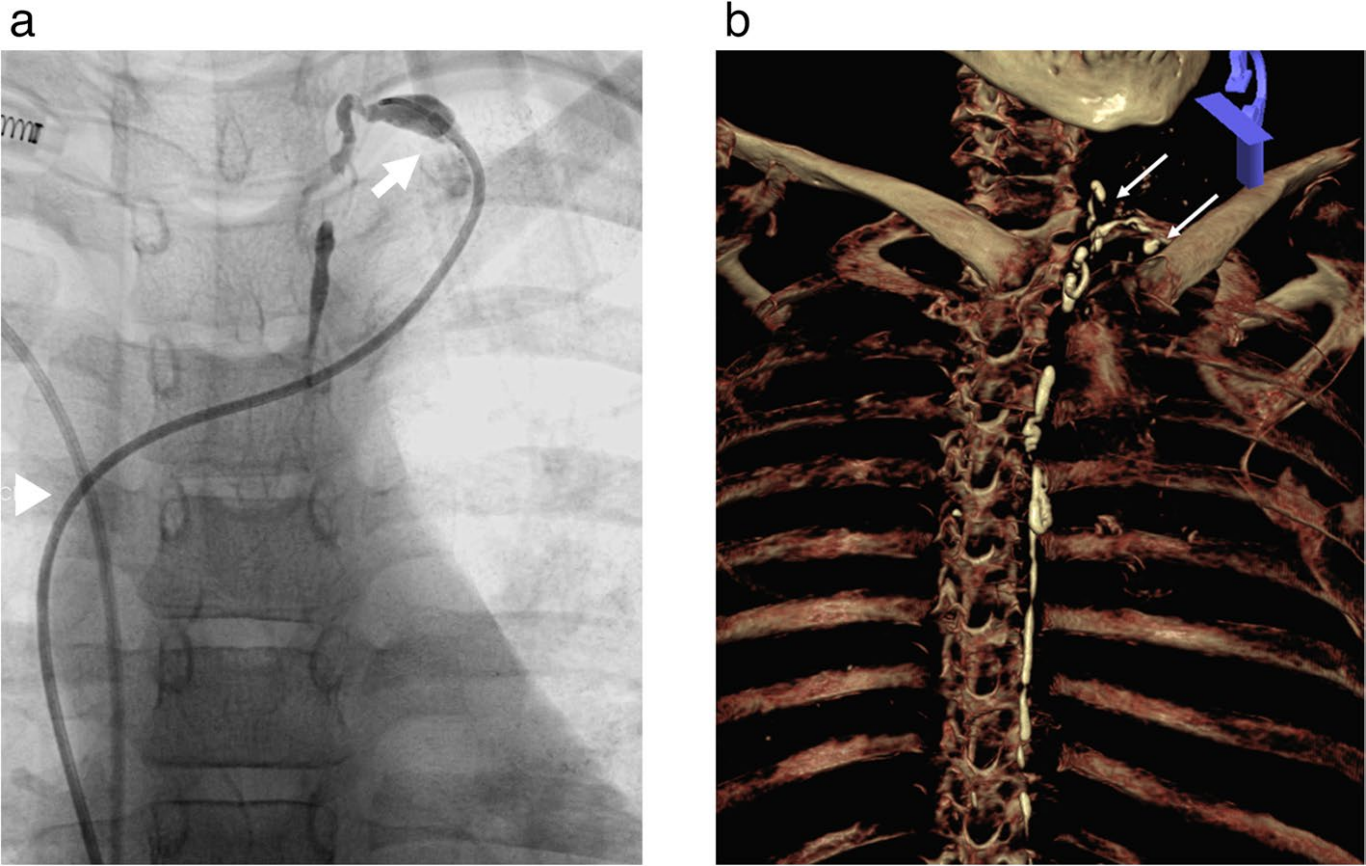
a, b Venolymphatic Anastomosis. a Retrograde catheterization with an angiographic catheter (arrow head) of the TD showing a single anastomosis (single arrow). b 3D Computed Tomography reconstruction in a TD junction showing multiple anastomosis (multiple arrows)
